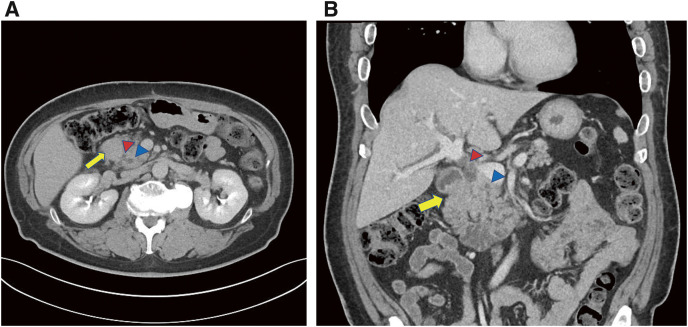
Computed tomography scan results at obstructive jaundice. (A and B) Computed tomography scan at readmission revealed duodenal wall thickening in the second portion of the duodenum (arrows) and dilation of the common bile duct (red arrowhead) and main pancreatic duct (blue arrowhead).
